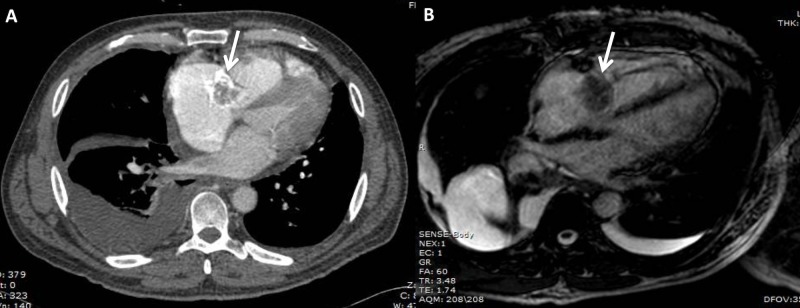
Further imaging with computed tomography (CT) and cardiac magnetic resonance (CMR). (A) Chest CT with a lobulated hypo-attenuating intracavitary right heart mass appearing to be centered in the tricuspid valve. (B) Cardiac magnetic resonance imaging showing no mass-vascularity.
